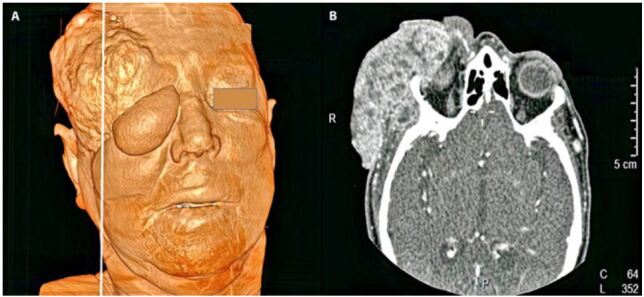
(A) A 3D tomographic image reconstruction showing an expansive lesion in the right frontal area with periorbital involvement. (B) Contrasted computed tomographic image showing an expansive lesion in the right frontal area, in the axial slideplane.
